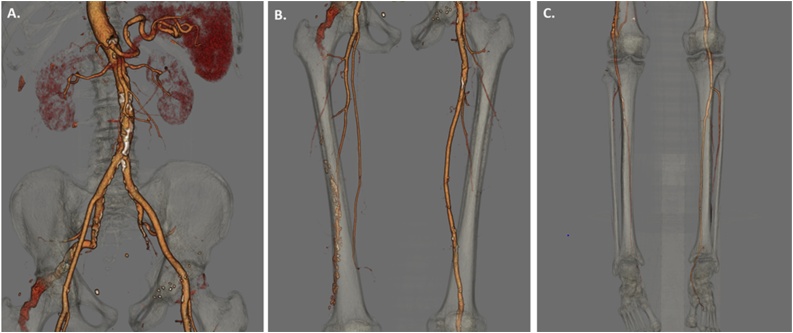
Computed tomography (CT) reconstructions demonstrating a right-sided persistent sciatic artery aneurysm. A) The right PSA is a continuation of the internal iliac artery and exits the pelvis caudally. B) The right PSA courses inferiorly along the entire right thigh. C) Continuation of right PSA as a popliteal artery. Note the hypoplastic nature of the distal right superficial femoral artery.
